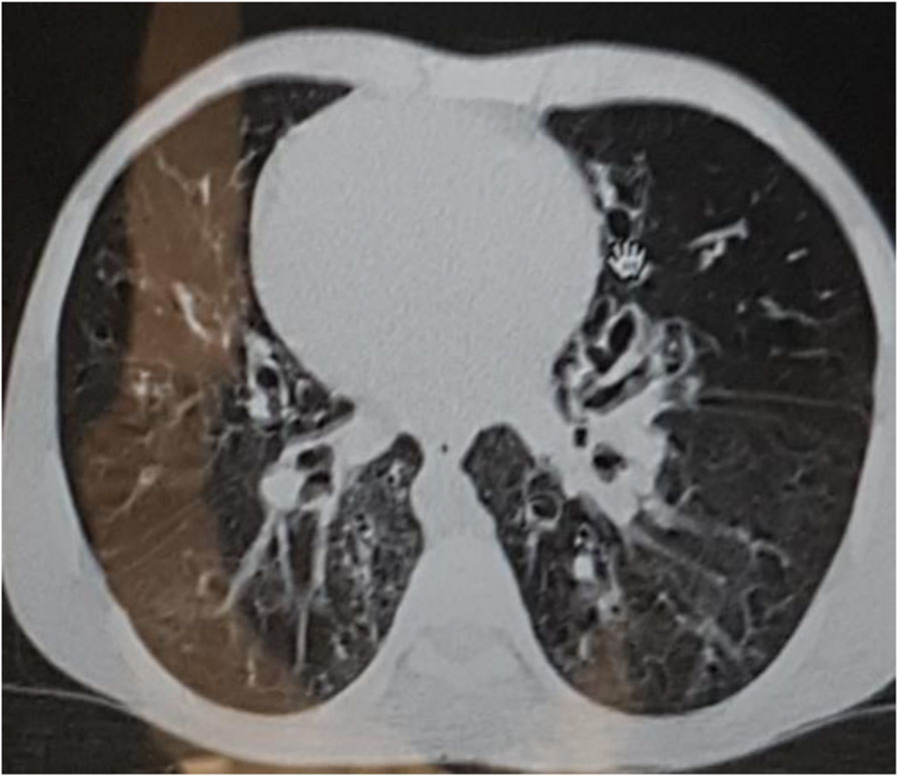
Axial post-contrast computed tomography showing cystic bronchiectasis on both lungs
